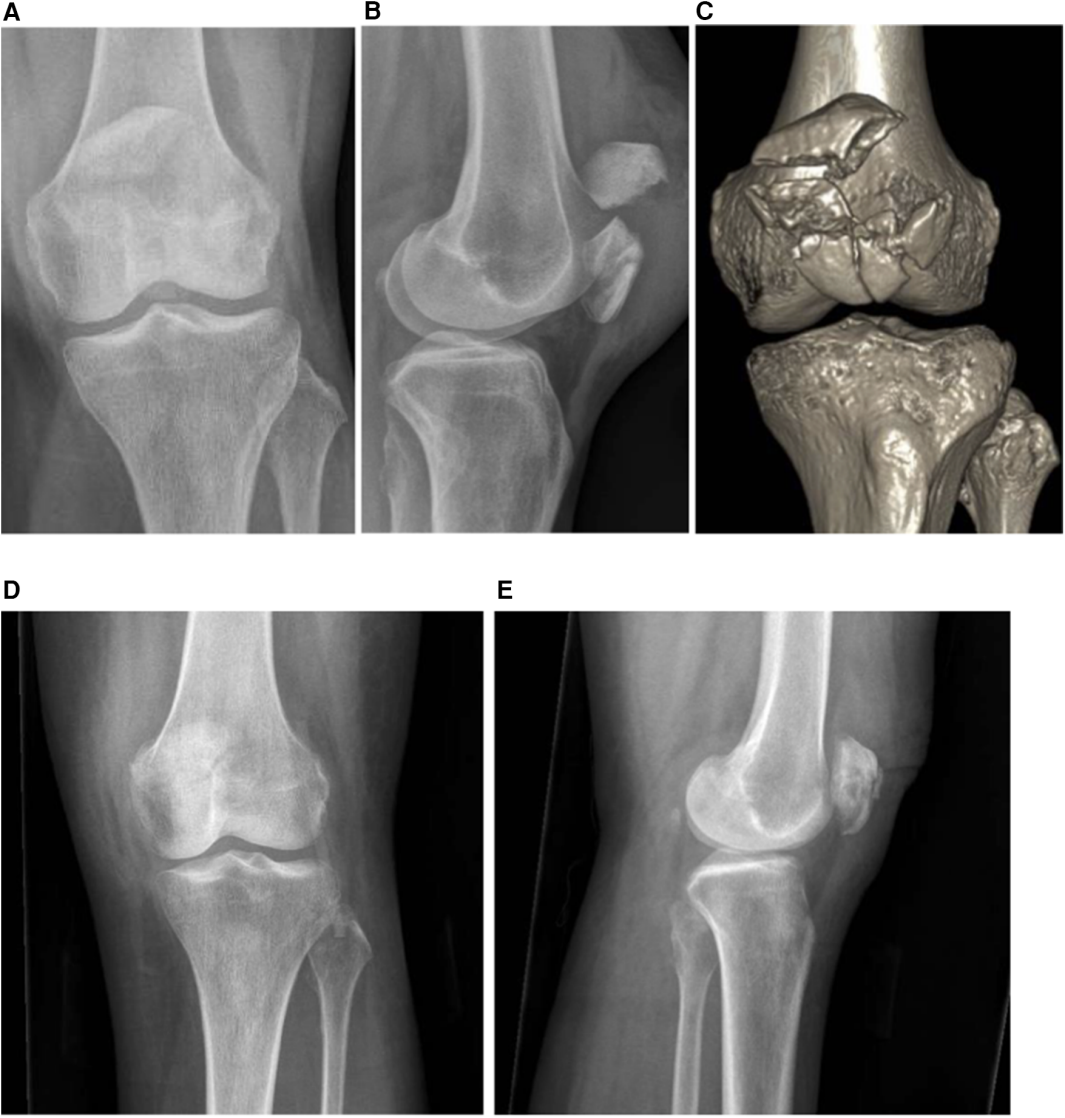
Case example of an AO34C3 patella fracture. (A,B) Anterior–posterior and lateral knee radiographs. (C) CT 3D reconstruction of the patella fracture. (D,E) Post-operative knee radiographs at the 3-month follow-up demonstrating good articular congruency attained.
